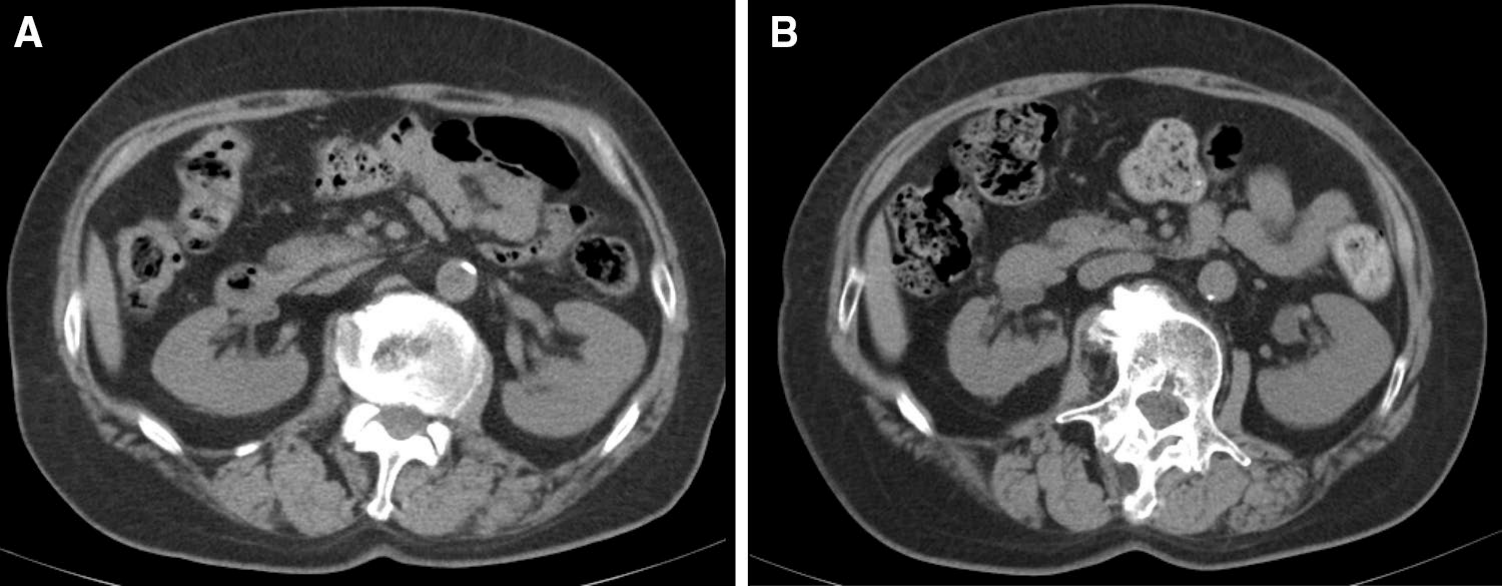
Plain CT images 10 months before (a) and on admission (b). The right renal parenchymal atrophy showed rapid progression during the 10-month period
